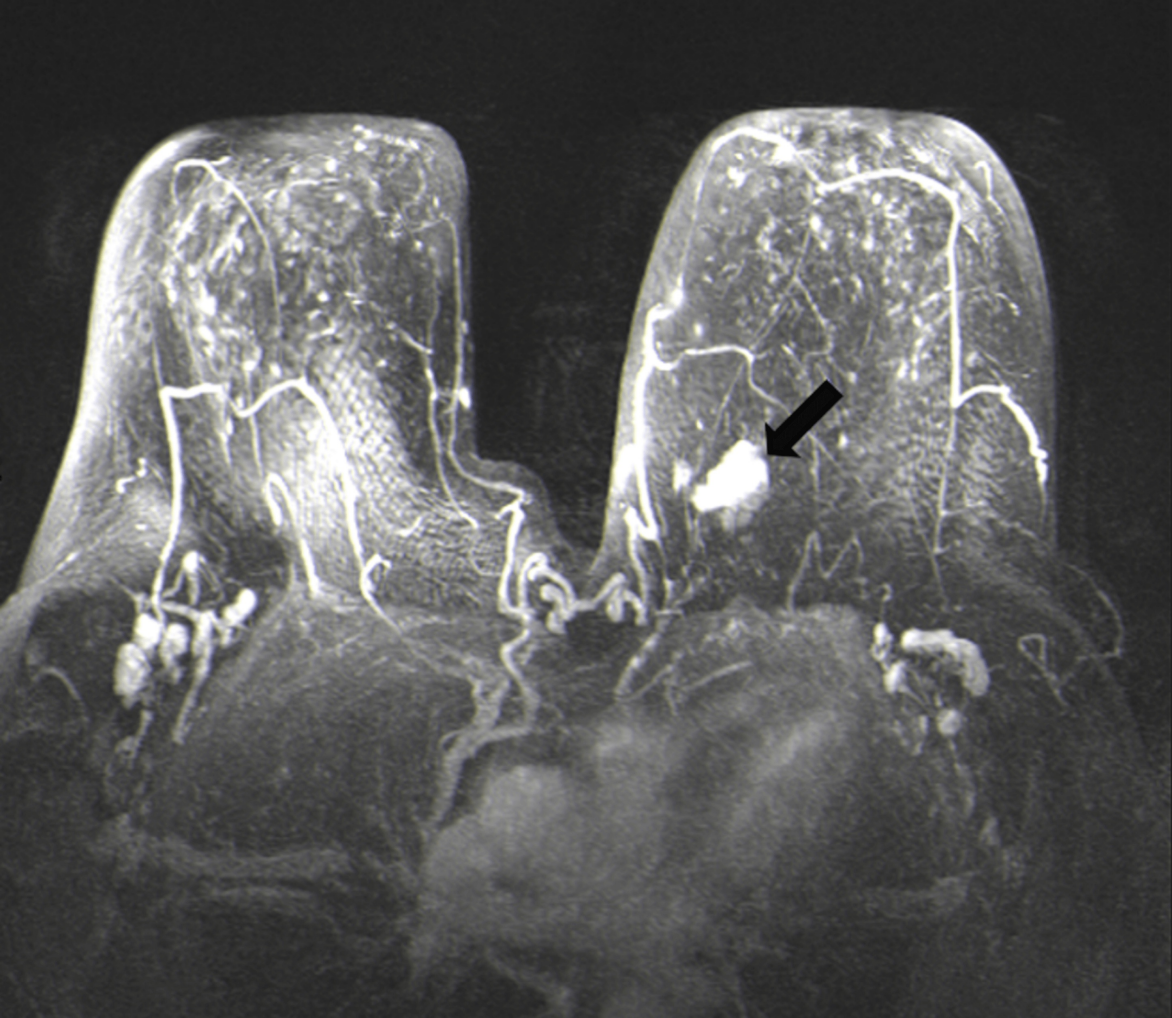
MRI of the breast showing a 27×20 mm solitary irregular lesion (arrow) in the upper inner quadrant of the left breast MRI: magnetic resonance imaging
